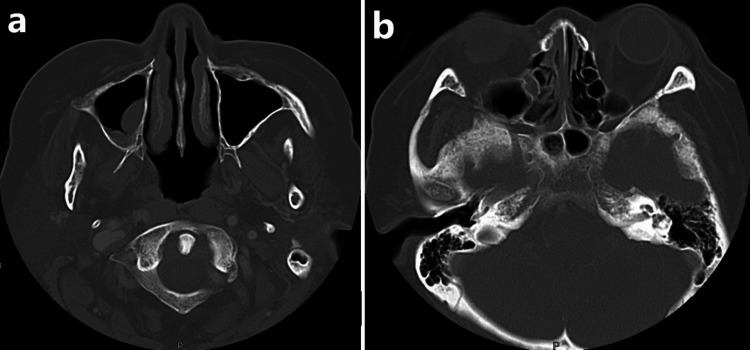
CT of the paranasal sinuses (axial view) revealed mucosal thickening of the right maxillary and sphenoid sinuses with surrounding sphenoid bone thickening
